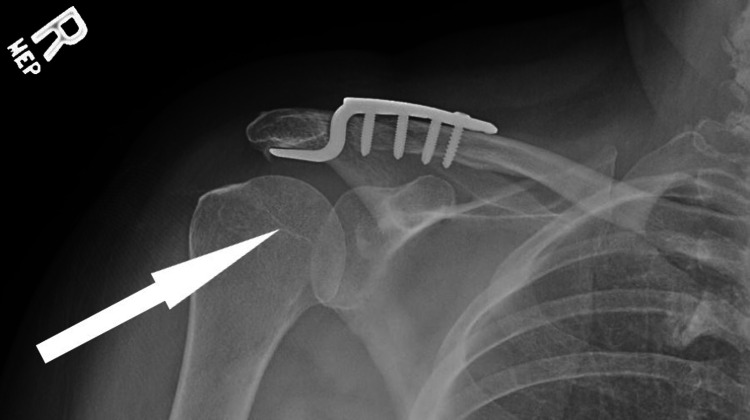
Post-procedural anterior-posterior (AP) X-rays demonstrating right acromioclavicular (AC) reconstruction using a DePuy Synthes hook plate DePuy Synthes, Johnson and Johnson
